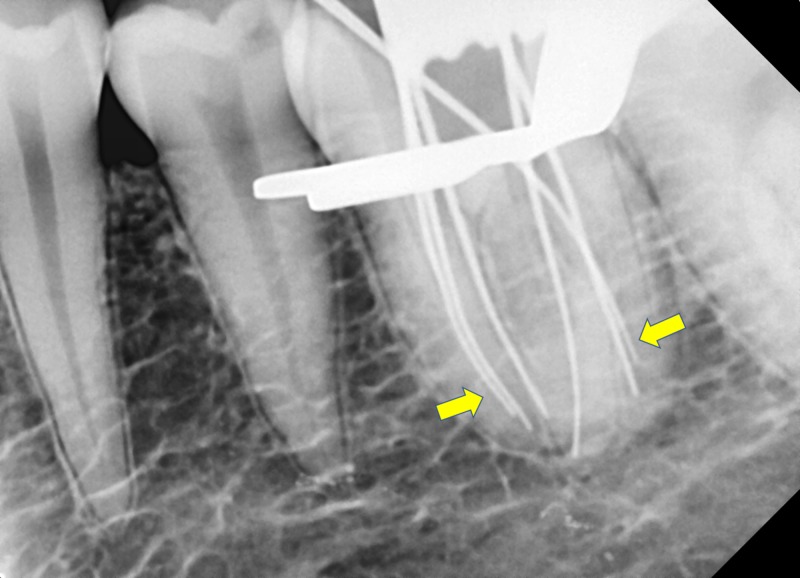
Working length radiograph showing six canals and extra distolingual root
